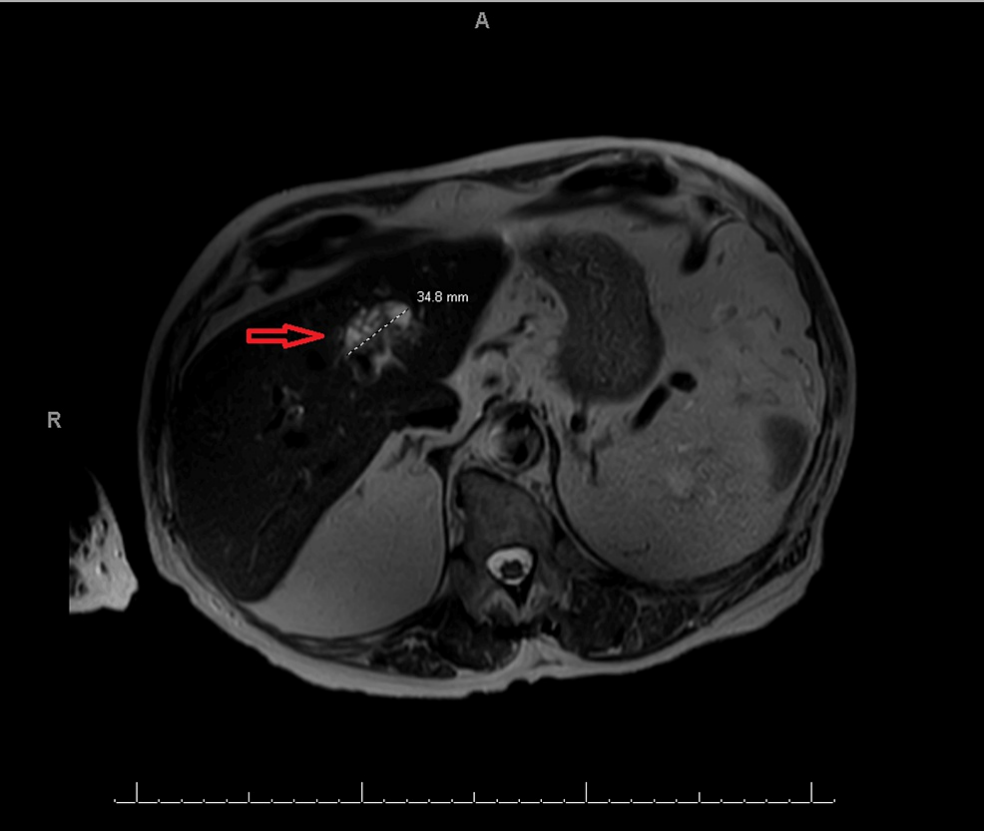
MRI of the abdomen showing an ovoid heterogeneous T2 hyperintense lesion (arrow) at the junctional segment II/IVa with mild extension into segment III/IVb with intrinsic heterogeneous and linear hypointense signal intensity.
